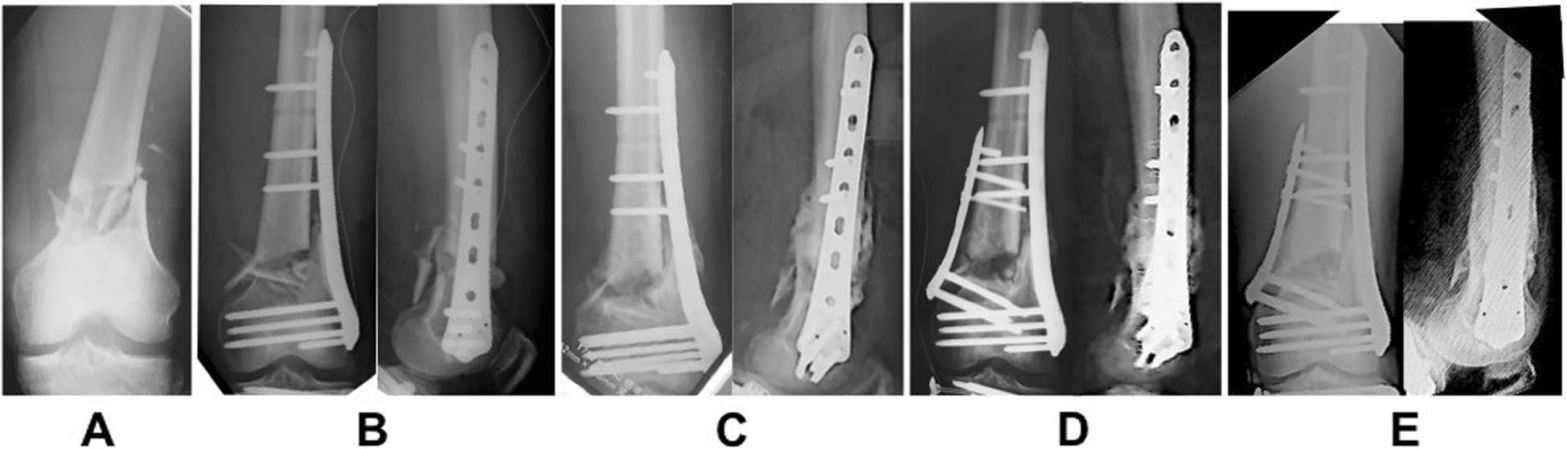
a Radiograph with Gustilo type-IIIA in a 73-year-old man. b Postoperative radiograph. c Radiograph and CT showed non-union 8 months postoperatively. d Another plate was added during re-operation to shorten the fracture. e Radiograph 1 year after re-operation
